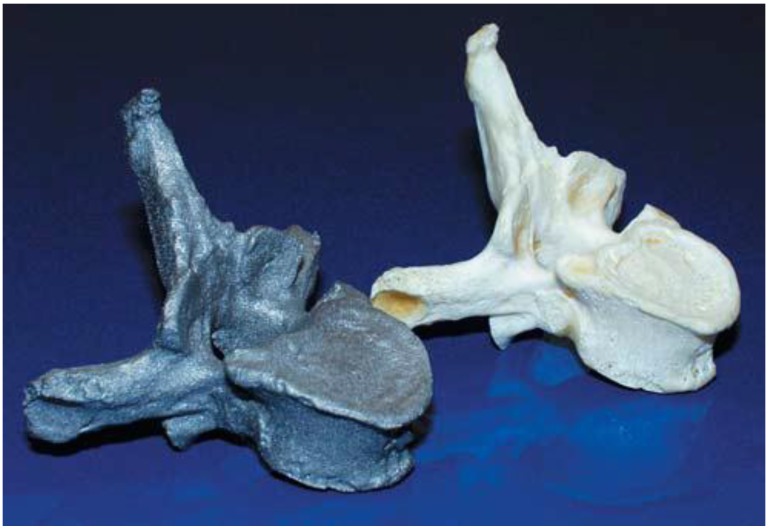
Example of an exact CT-based part of a complex human vertebra processed by SLM using additive manufacturing [37].
